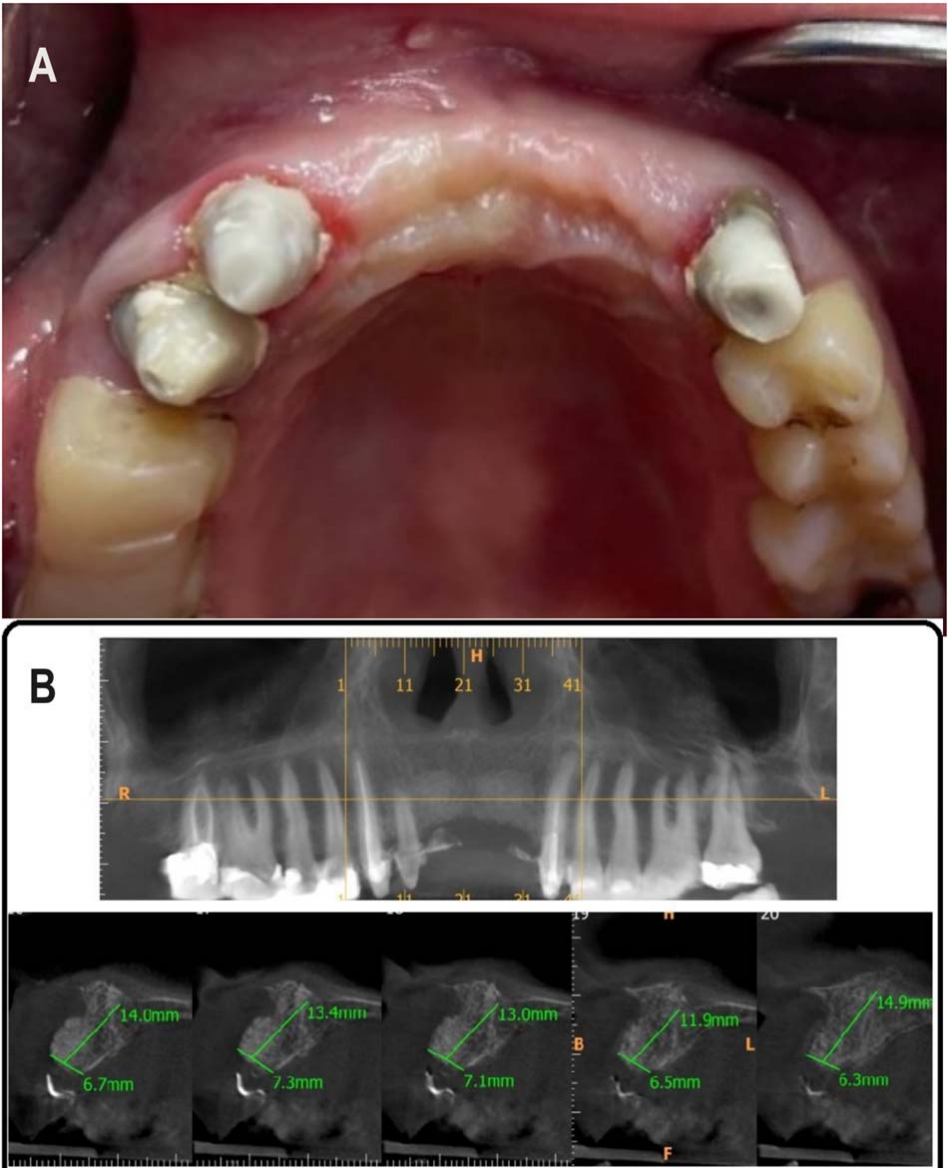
(A) 5 months postoperative photograph, (B) 5 months postoperative CBCT.
